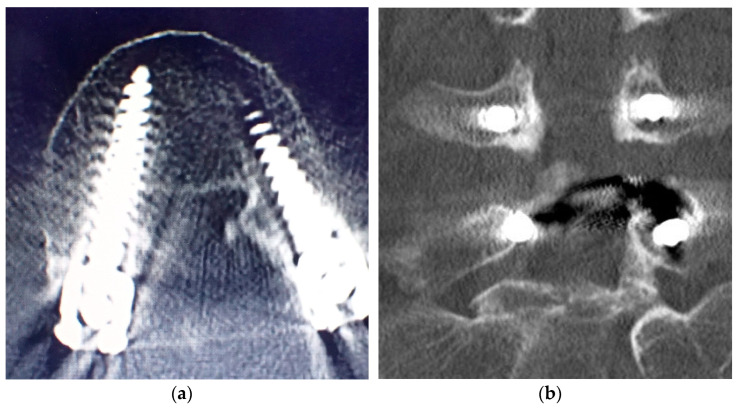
(a,b) Computed tomography scan of the screw deviation to the medial pedicle wall in grade D breach.
